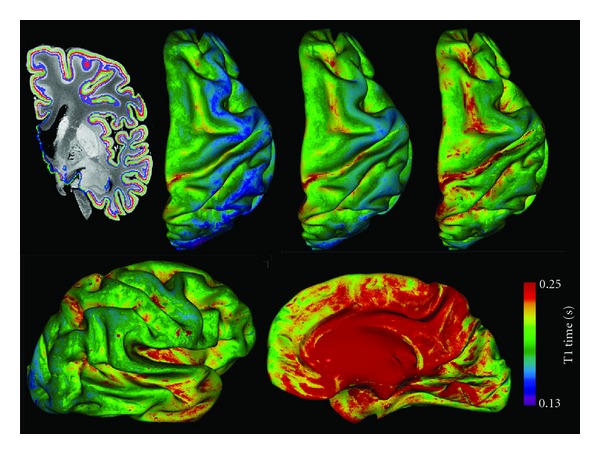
Top row: T1 times mapped onto inflated cortical contours extracted at 75%, 50% and 25% cortical depth (left to right) from the pial surface. The contours are outlined on a coronal view of an MR image in blue, red and green respectively. Bottom row: lateral and medial view of the T1 times at 25% cortical depth.
